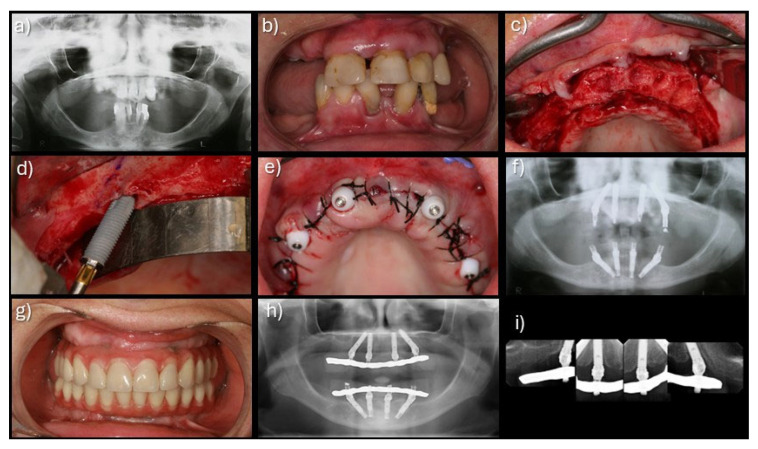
Clinical case of an immediate implant-supported full-arch maxillary rehabilitation using 4 implants and posterior implant tilting, from implant insertion to 20 years: (a) pre-treatment orthopantomography; (b) pre-treatment intra-oral view; (c) per-operative intra-oral view after teeth extraction and bone regularization; (d) per-operative intra-oral view of the insertion of the tilted posterior implant anterior to the maxillary sinus; (e) per-operative intra-oral view after implant insertion and suturing; (f) orthopantomography on the day of surgery with immediate prosthesis in place achieving immediate function; (g) intra-oral view of the definitive prostheses in place; (h) orthopantomography with the definitive prostheses in place; (i) periapical radiographs of the maxillary rehabilitation at 20 years of follow-up.
